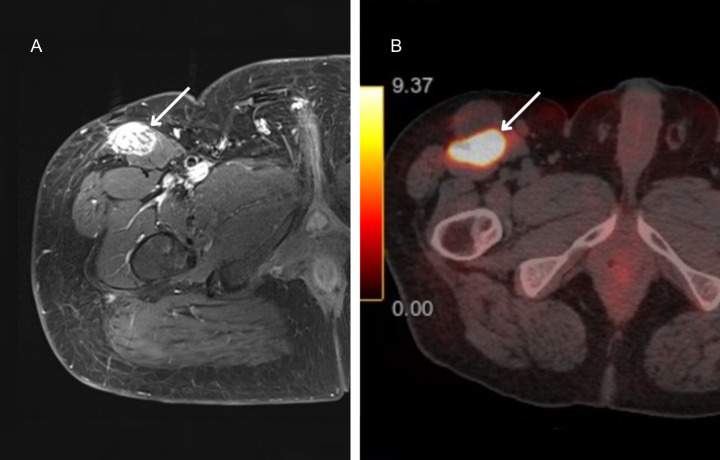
Imaging evaluation of the tumor: (A) Axial magnetic resonance imaging of the right thigh demonstrating a heterogeneous, well-circumscribed mass measuring 47 × 40 × 24 mm in the proximal anterolateral portion of the sartorius muscle with intense, heterogeneous post-contrast enhancement (white arrow). (B)^68^Ga-DOTA-TATE PET/CT demonstrates intense radiotracer uptake (42 × 41 mm; SUVmax 33.2) within the proximal right thigh lesion, with no evidence of distant metastatic disease (white arrow).
